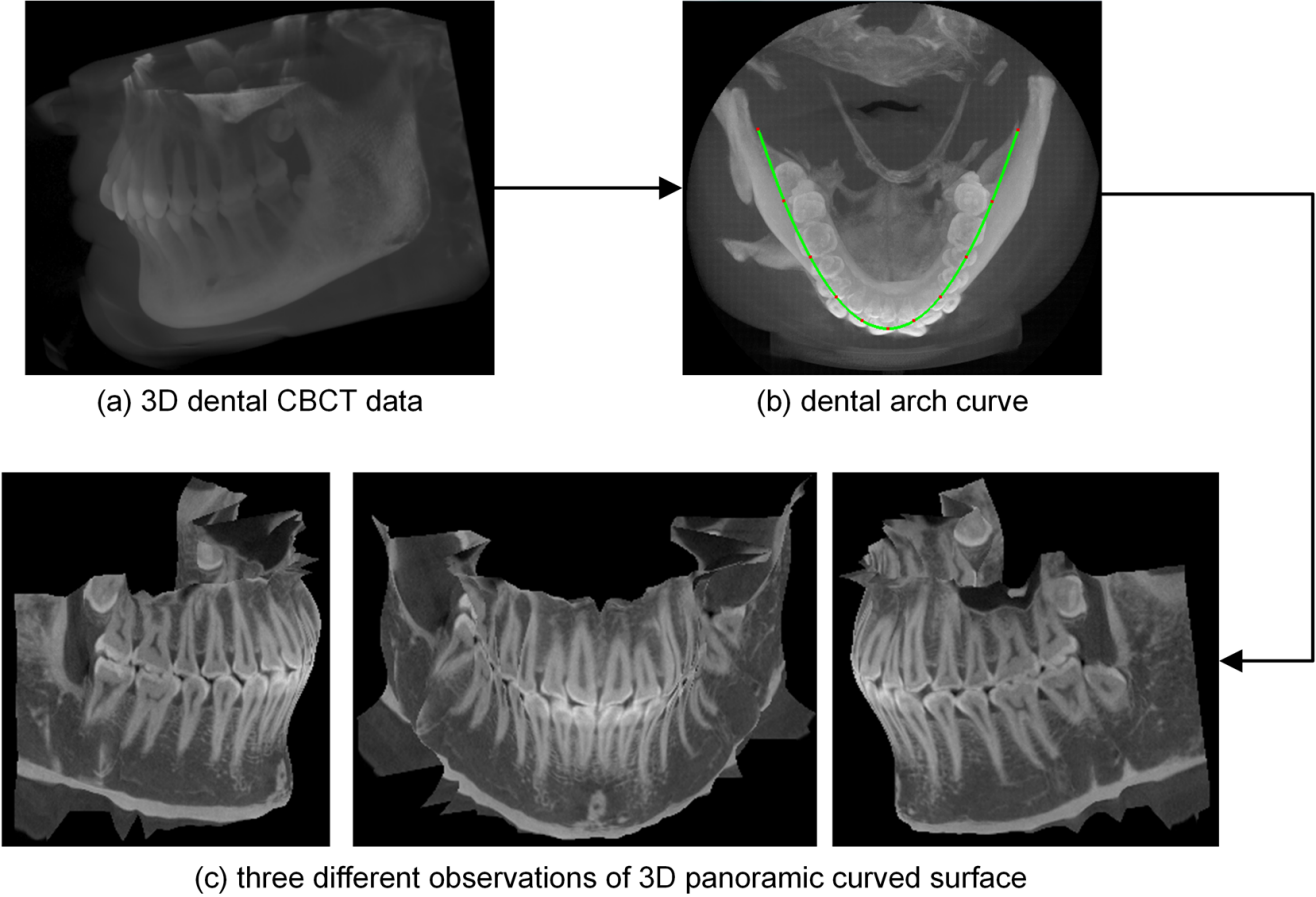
Results of our method from the closed-bite shaped dental CBCT dataset 2.
